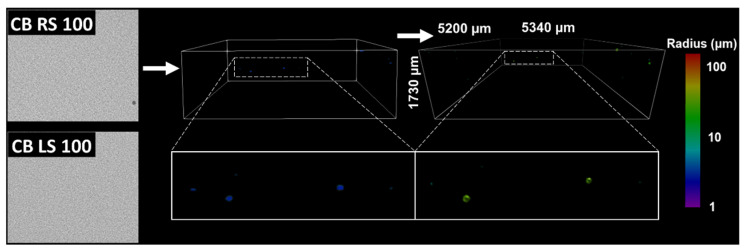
Pore network visualizations for 100 s^−1^ cocoa butter samples. (Left) CT images slices, (Middle) raw 3D plots (blue) of CB RS 100 void and (Right) a 3D ball and stick plot of CB RS 100 pores and throats after MB analysis. No 3D visualizations for CB LS 100 were created because these networks have no voids.
